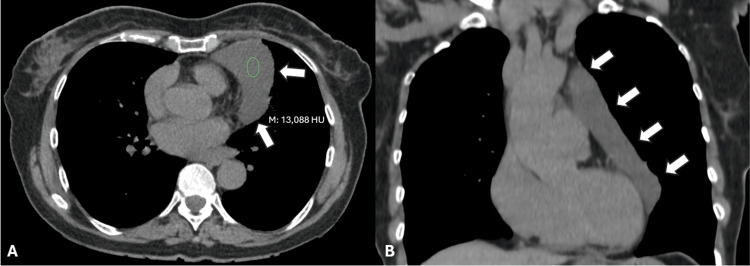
(A) Soft tissue window axial non-contrast chest CT revealing a large, well-circumscribed, homogeneous lesion with fluid attenuation (medium density of 13 HU), adjacent to the pericardium (white arrows). (B) Soft tissue window coronal non-contrast chest CT showing the fluid attenuation lesion, with extension to the aortic arch (white arrows). Fat planes with the heart chambers and aortic arch can be seen. CT: computed tomography, HU: Hounsfield unit
